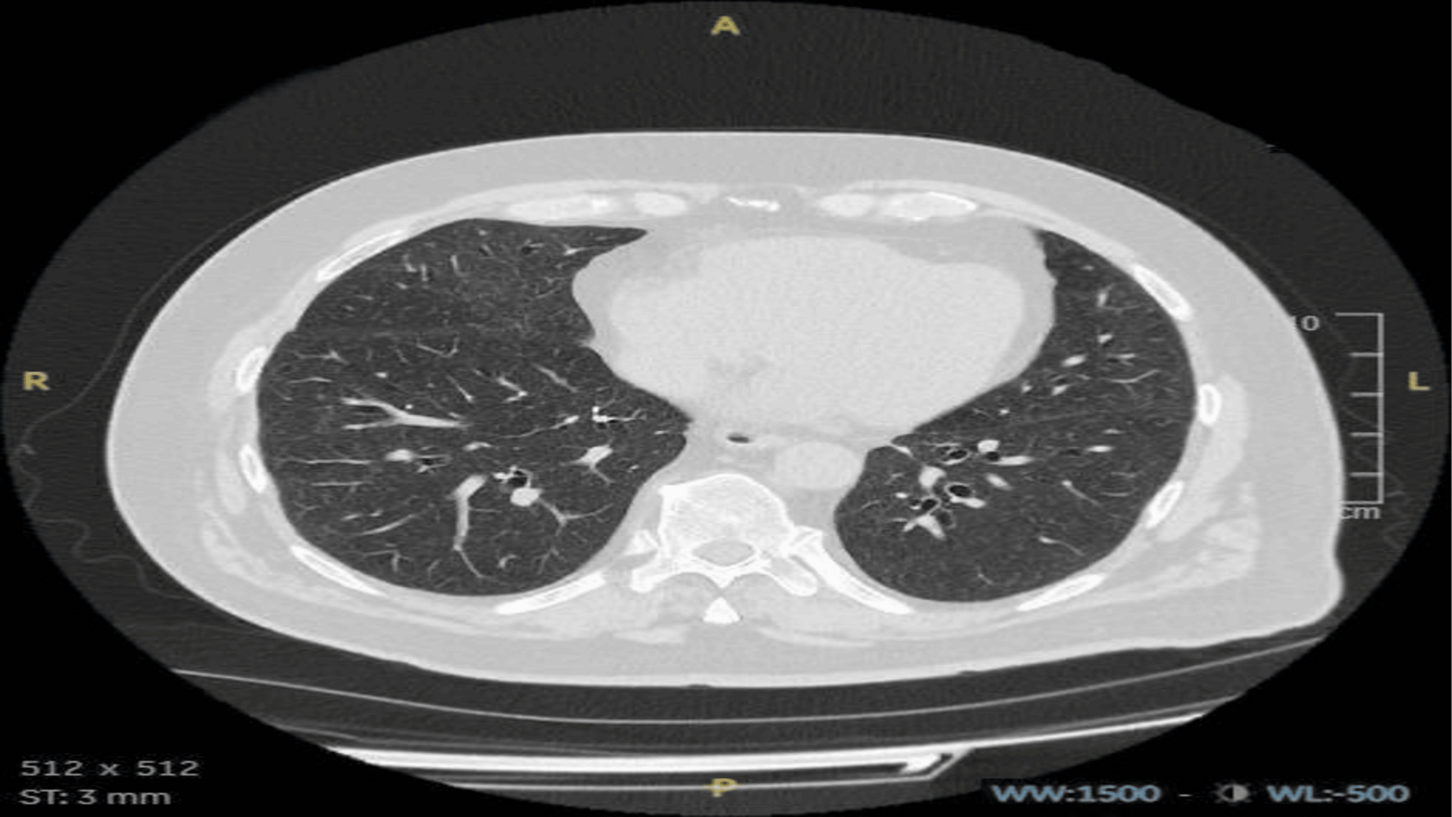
Repeat chest CT three months post-admission Repeat chest CT performed three months post-admission, following avoidance of hot tub exposure, showing significant resolution of previously observed ground-glass opacities and improving nodularity. CT, computed tomography
